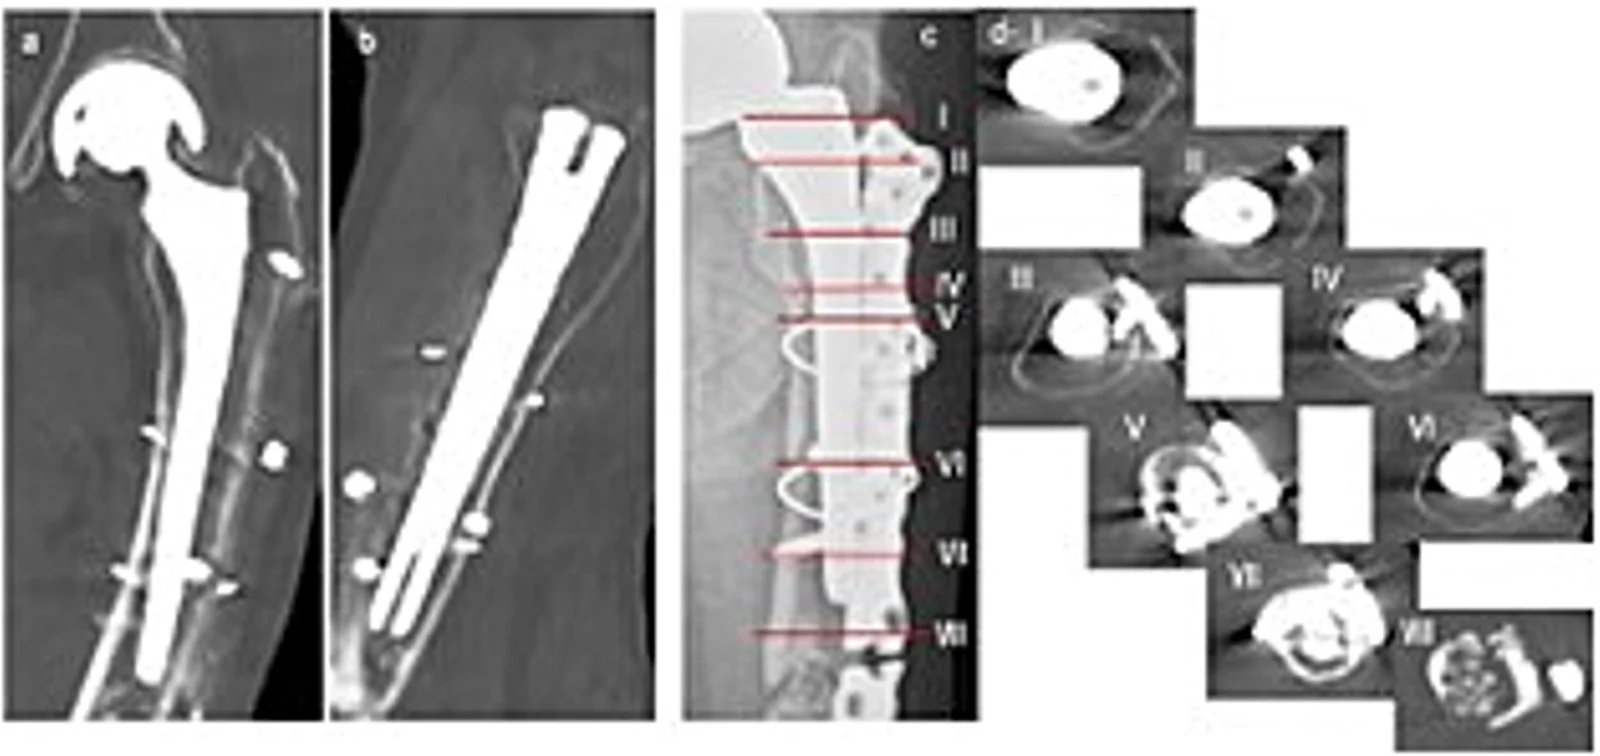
Plain CT imaging. (a) Coronal reconstruction, (b) sagittal reconstruction, (c) scout view, and (d) axial CT images at the levels indicated in (c) (d-I to d-VIII). These images demonstrate no circumferential gap between the stem and femur from proximal to distal. Marked cortical thinning of the proximal femur attributable to stress shielding is also evident.
